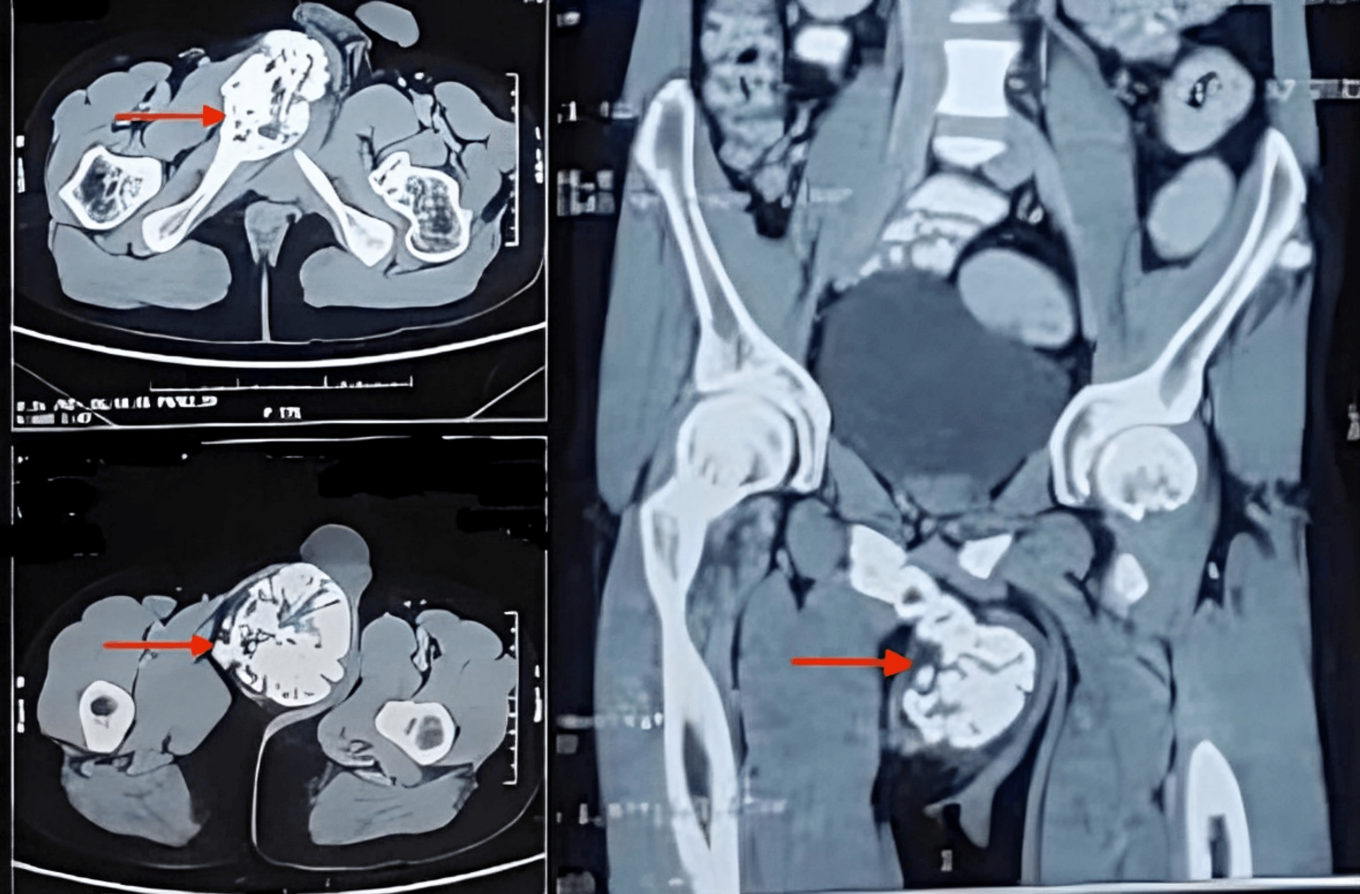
Computed tomography images Axial view (left) and coronal view (right) of the pelvis showing right ischiopubic ramus osteochondroma.
